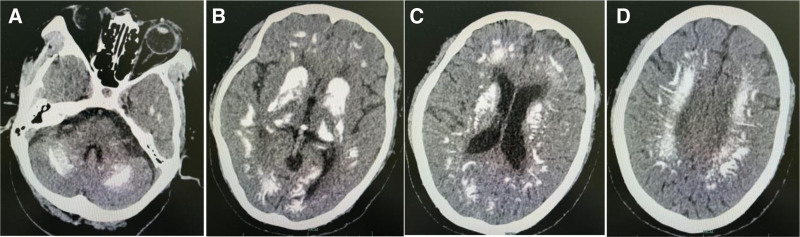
Brain CT showed bilateral symmetrical calcification: (A) cerebellum; (B) corpus striatum, thalami and occipital lobe; (C) frontal lobe and paraventricular white matter; (D) centrum semiovale of both cerebral hemispheres.
